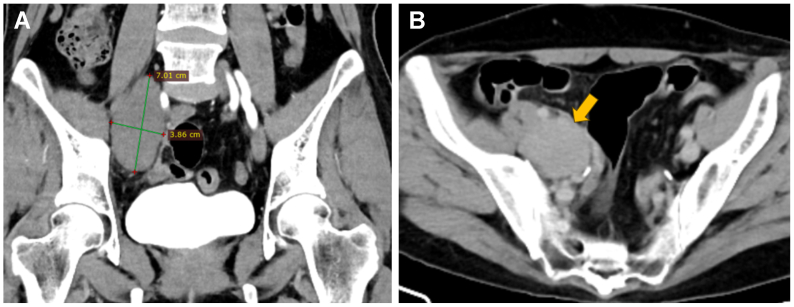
Contrast-enhanced computed tomography scan. (A) Coronal reconstruction reveals a large space-occupying lesion contiguous with the right external iliac vein. (B) Axial view during the venous phase demonstrates mild enhancement of the aneurysm (*arrow*).
